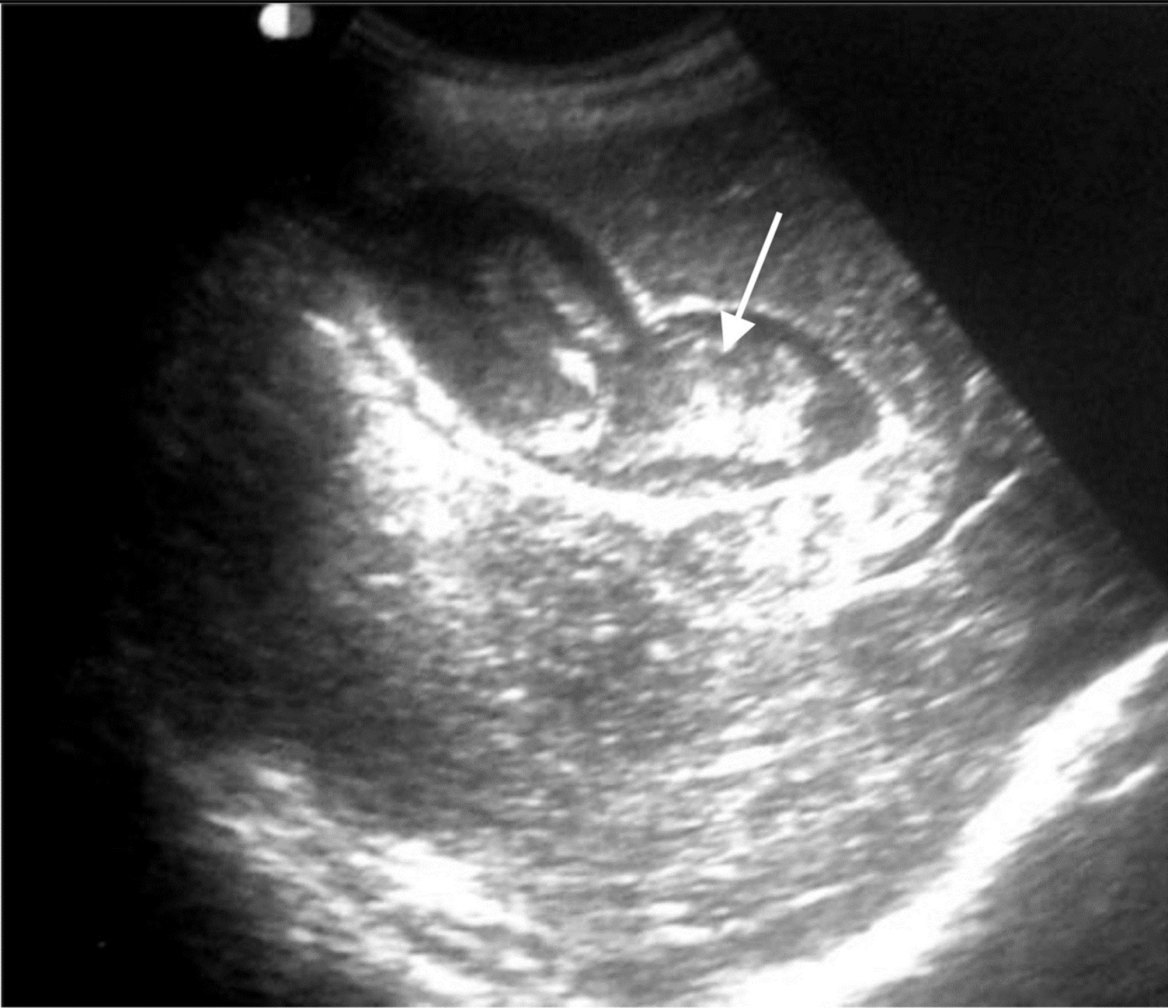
Ultrasound examination. The gallbladder showed an echogenic blood clot (arrow) in the lumen.
